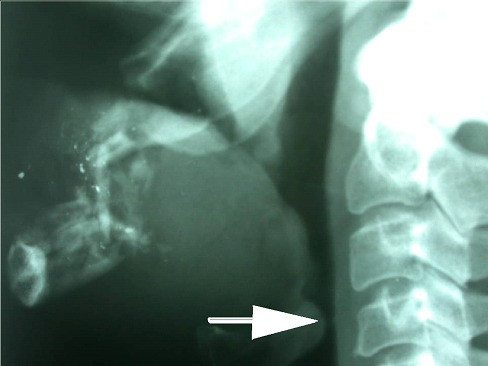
Male patient who sustained high velocity injury to the lower face. Tracheostomy was performed in the Shock-Trauma Unit. Lateral x-ray shows comminuted fracture of the mandible with huge soft tissue swelling of the neck and narrowing of the airway (white arrow).
